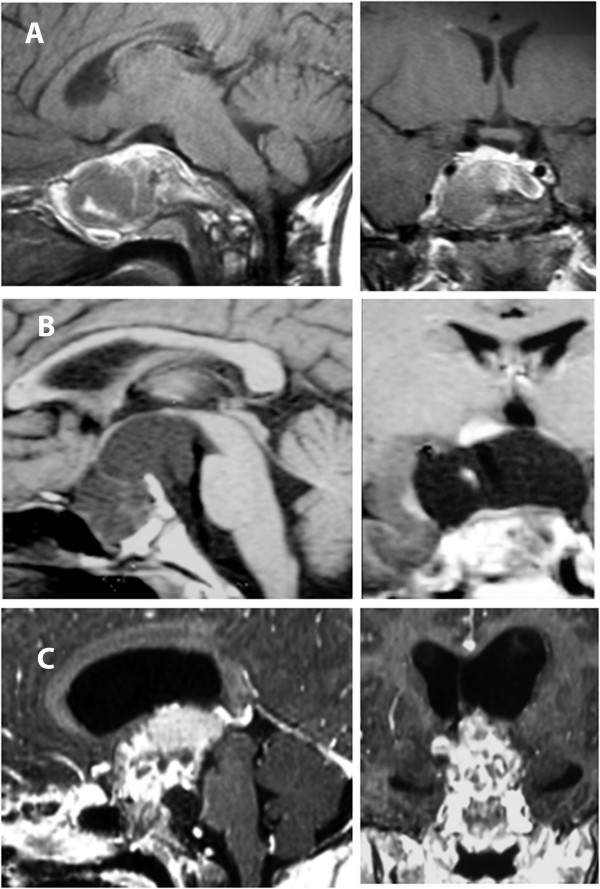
Enhanced T1 weighted MRI's of craniopharyngiomas. a. Apredominantly solid sellar/supra sellar tumour that is discrete fromthe hypothalamus (grade 0). b. A predominantly cystic sellar/suprasellar tumour that is distorting but not invading the hypothalamus (grade 1). c. A predominantly solid sellar/supra sellar tumour. The hypothalamus is not visible because of tumour invasion (grade 2).
